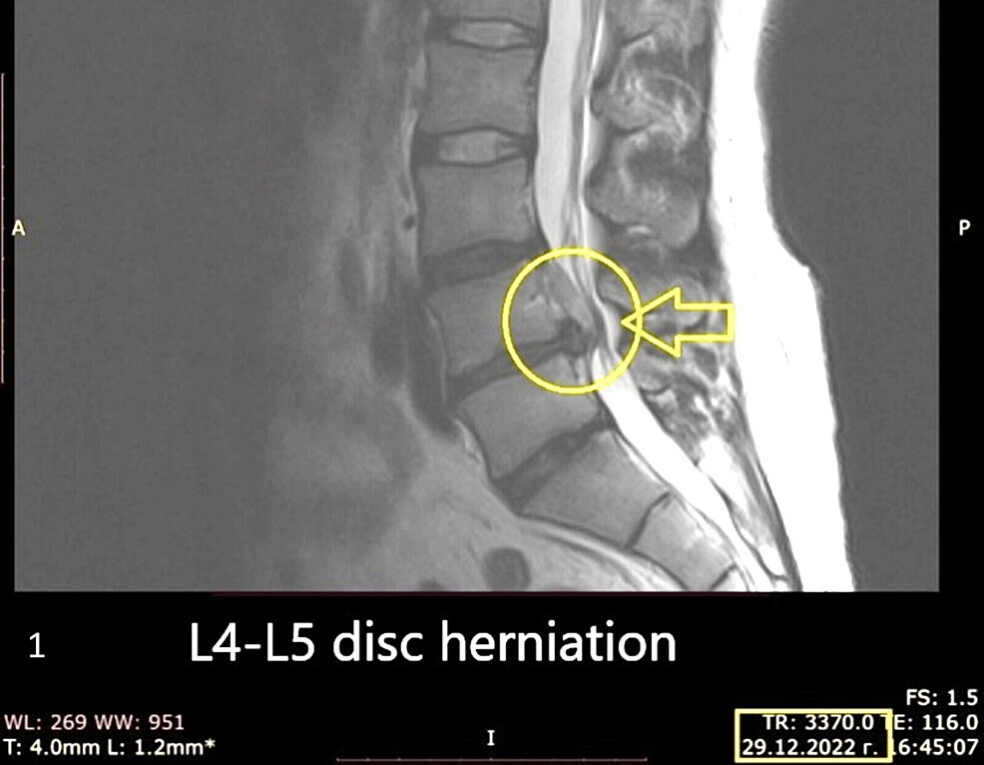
An MRI (sagittal plane) found evidence of an L4-L5 disc herniation (Date: 29.12.2022).
